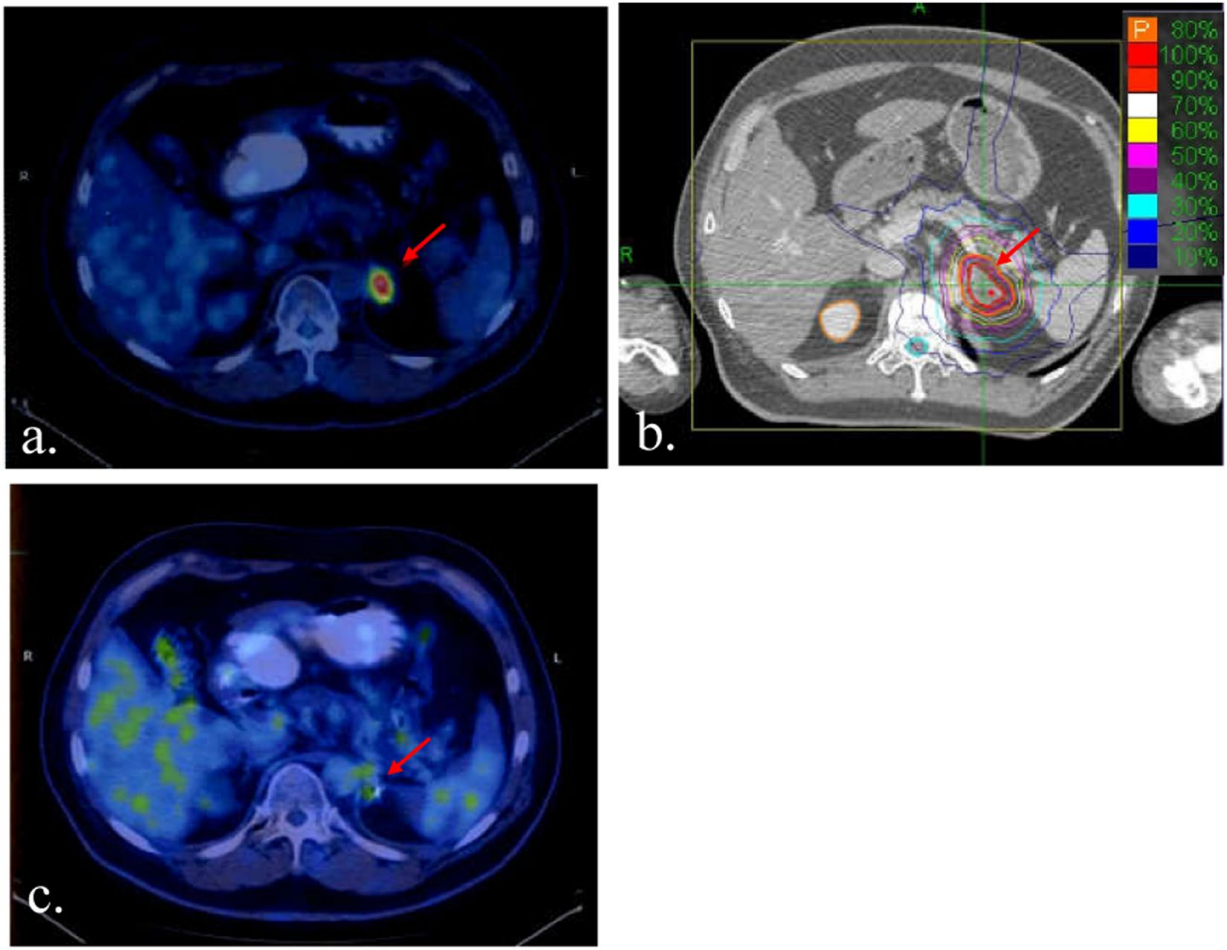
Example of dose distribution and treatment outcome. (a) PET-CT scan before SBRT. The arrow shows that the SUVmax of the adrenal metastasis is 8.4 (b) Treatment plan with 45 Gy in 3 fractions. The arrow shows that the isodose line is 80% (c) PET-CT scan three months after SBRT. The arrow shows no radioactive concentration in the lesion after SBRT.
